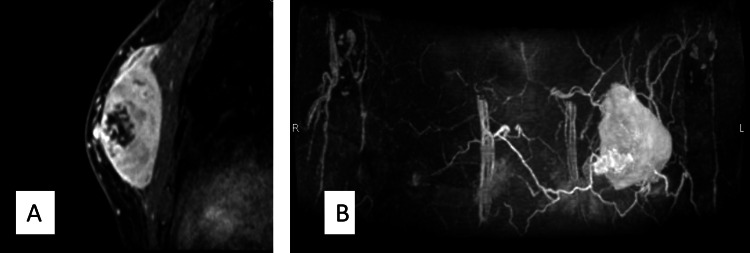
MRI of the patient (A) A 68 x 34 x 84-mm large-sized tumor with low signal intensity in fat suppression T1-weighted images (B) High signal intensity in T2- and diffusion-weighted images, and low values in an ADC map ADC: Apparent diffusion coefficient
